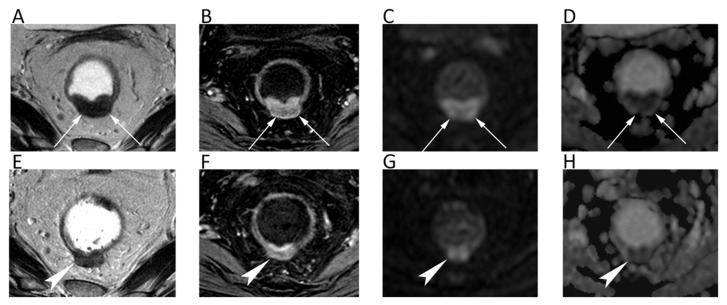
MRI scans of a 66-year-old female patient with rectal cancer at the initial diagnosis and two months later after neoadjuvant chemotherapy. Axial T2w image (A) and axial post-contrast fat-suppressed 3D GRE T1w image (B) show the tumor along the posterior rectal wall (arrows) with high signal intensity on the axial *b* = 600 DWI image (C) and low ADC (mean ADC: 0.651 × 10^−3^ mm^2^/s) on the corresponding ADC map (D). Post-treatment axial T2w image (E) shows the reduction in size of the lesion (arrowheads) with decrease of contrast enhancement (F) and slight increase of ADC values (G,H).
